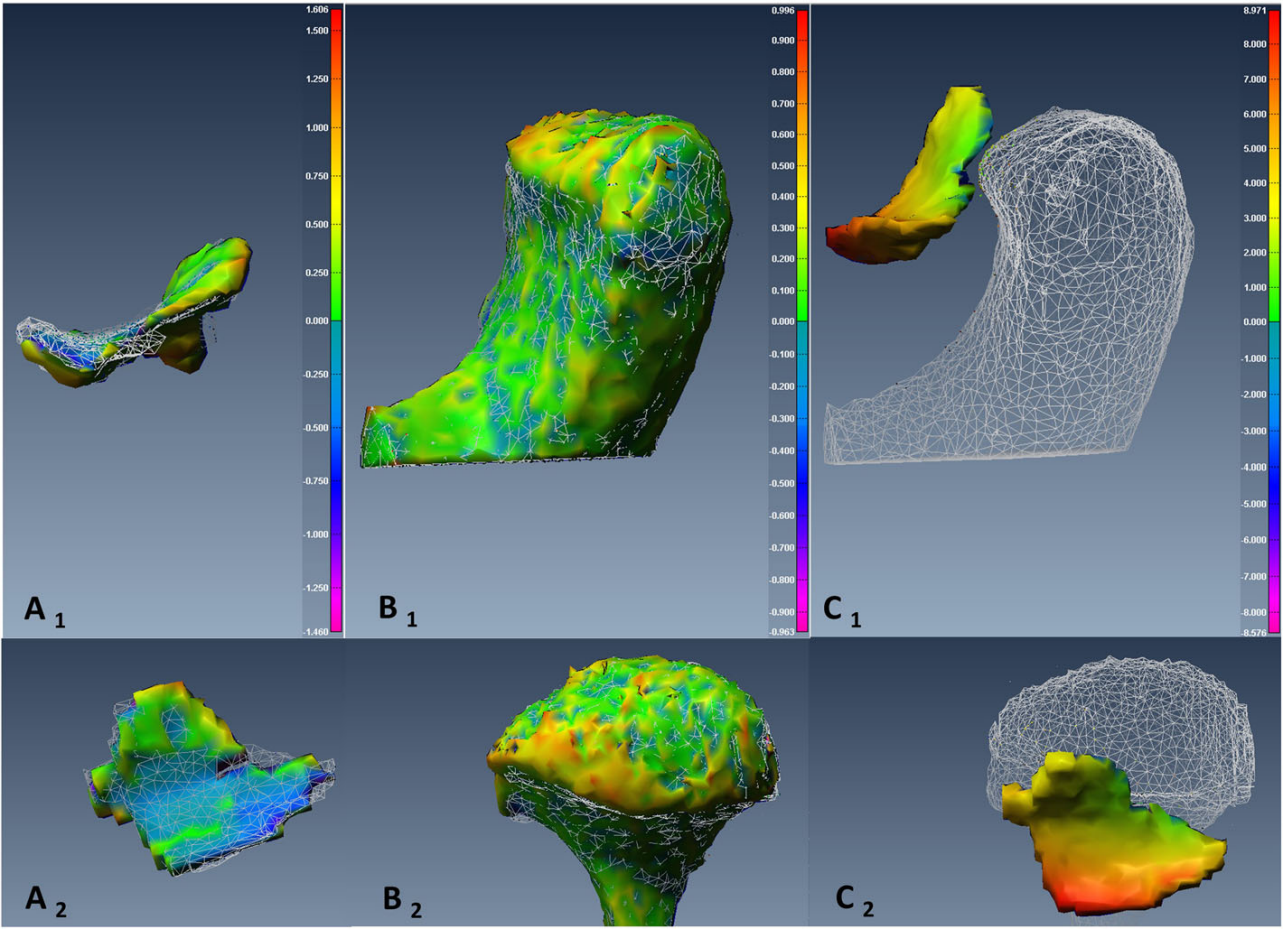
TMJ 3D reconstructed models representative of TMJ from an MRI-CBCT co-registered image of subject number 3 pre- and post-transoral surgery. The TMJ showed small change in disc and condyle positions between pre- and post-surgery. *A*
